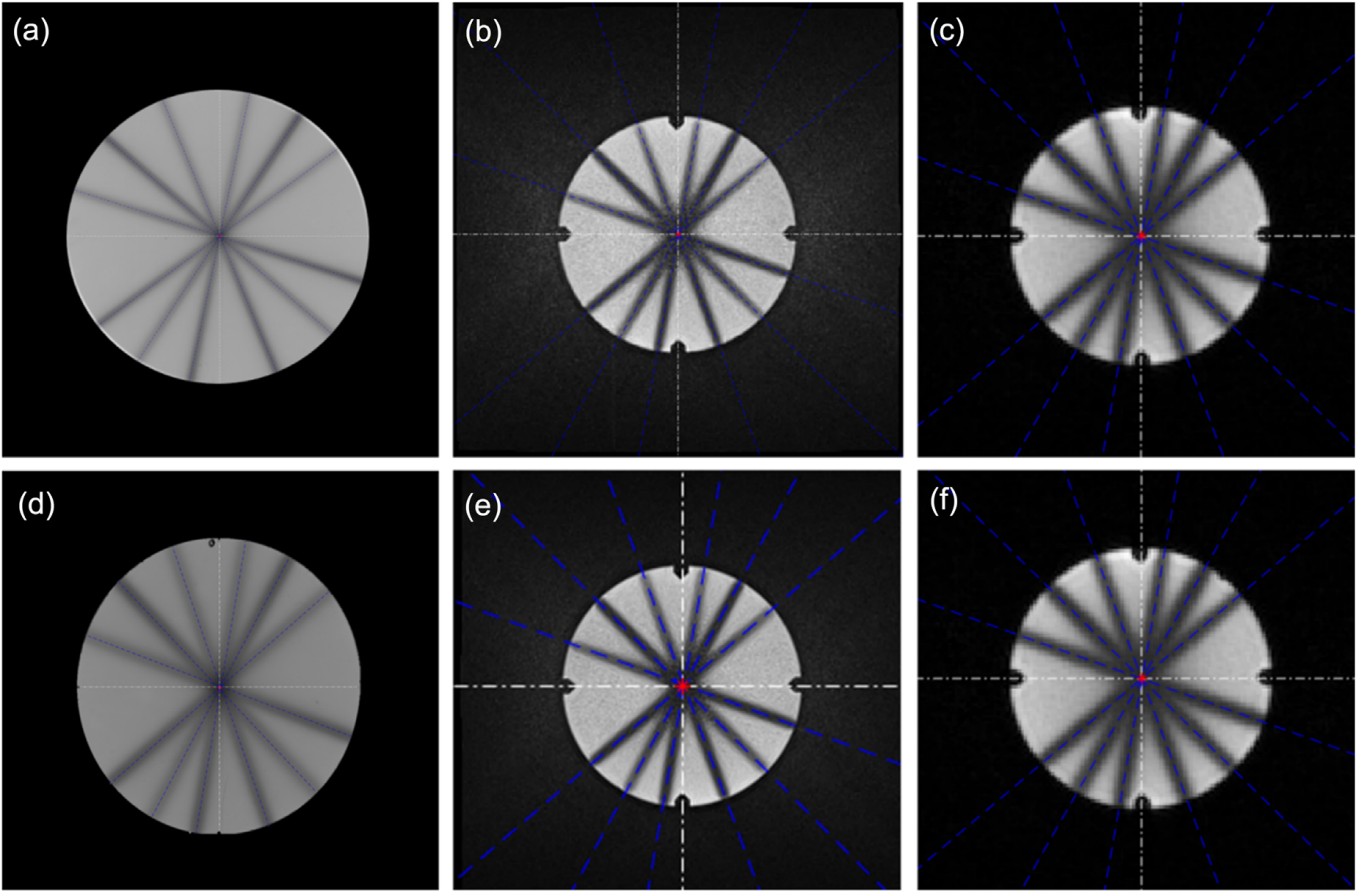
(a) Scanned image of EBT3 film, (b) MR image with T2‐w, and (c) MR image with TrueFISP of MAGAT gel for Linac; (d) scanned image of EBT3 film, (e) MR image with T2‐w, and (f) MR image with TrueFISP sequence of MAGAT gel for MR‐Linac. The blue dashed line is central line for each angle. The white dashed line is imaging isocenter and the red circle is isocircle
